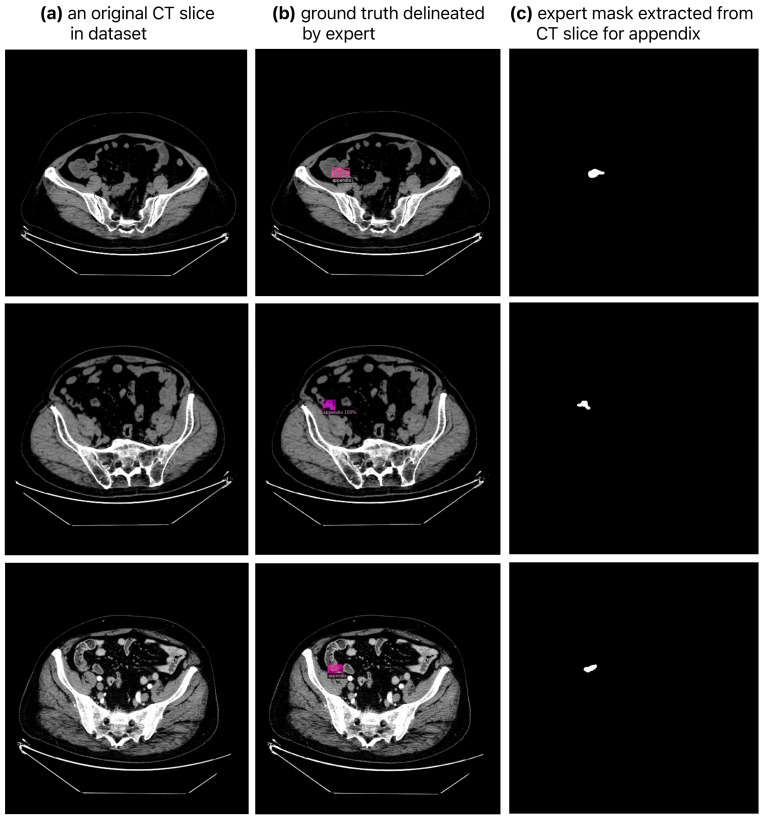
Original CT in dataset slices and the appendix region masks in these CT slices extracted by expert physicians.
